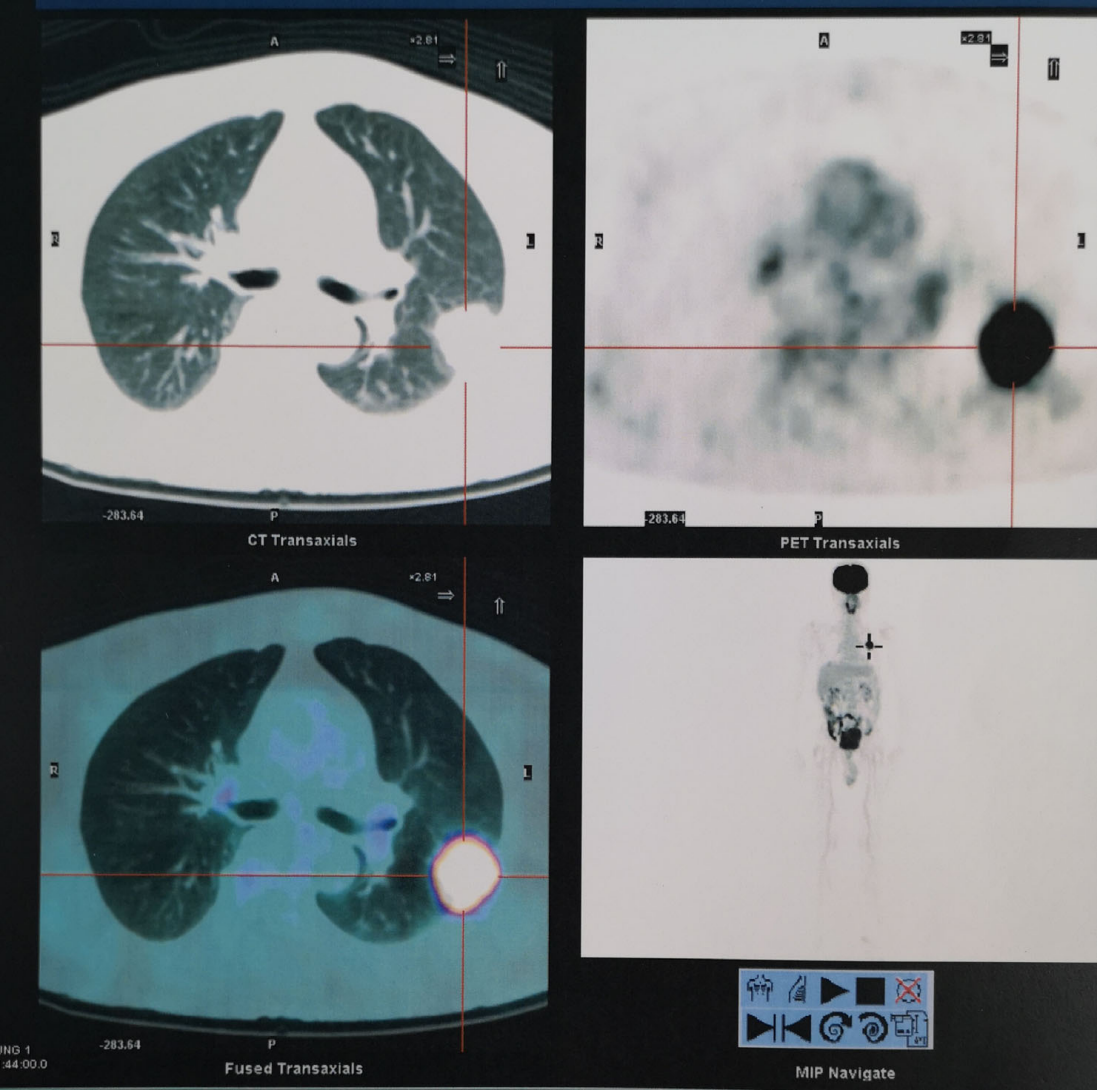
Positron emission tomography of the thorax. A lobulated FDG‐avid mass was seen at the left upper lobe (SUVmax: 20.2) with a metabolic size of 3.7 × 3.0 × 3.8 cm. No other FDG‐avid nodule/mass was seen at the remaining left lung parenchyma or at the right lung parenchyma. No FDG‐avid nodal metastasis or evidence of distant metastasis was seen.
